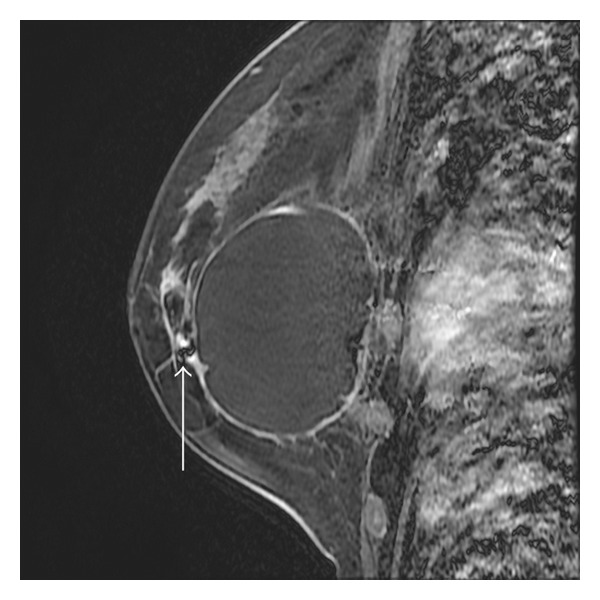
Sagittal postcontrast breast MRI of an augmented breast with silicone injection and mammoplastic bag placement showed a 0.8 cm lobular mass beneath the augmented mammoplasty bag, with placement of an MRI-compatible guide wire (white arrow) for excision localization.
